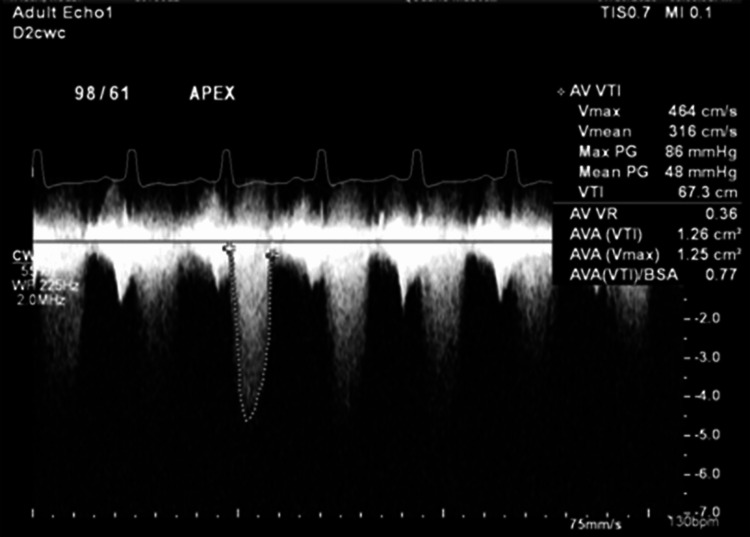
Continuous wave Doppler through the aortic valve, emphasizing a severely elevated mean pressure gradient and velocity
